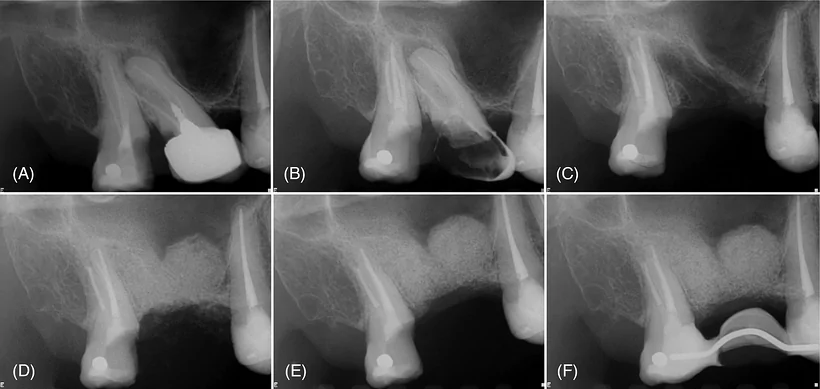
Case 3: Periapical radiograph showing a radiolucent lesion affecting the periapical areas of teeth #1 and #2, interproximal caries, defective restorations, and unsatisfactory root canal treatments (A). Periapical radiograph after root canal retreatment of tooth #1 (B). Intraoperative radiograph following the extraction of tooth #2, alveolar curettage and apicoplasty of tooth #1 (C). Immediate postoperative radiograph after bone regeneration and sinus lift (D). Radiographs at the 1‐month (E) and 3‐month (F) follow‐ups.
